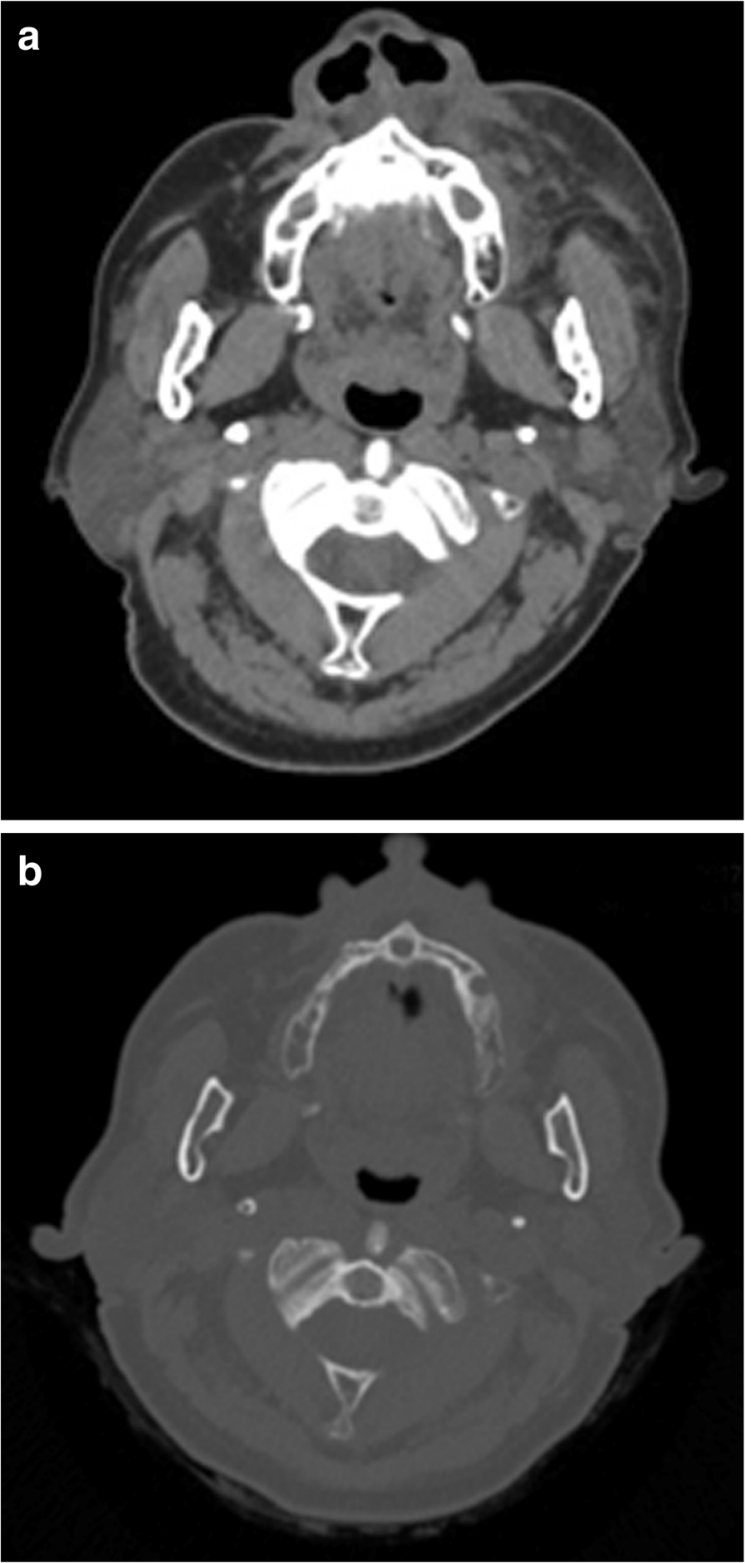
Simple computed tomography image of the neck at the first examination. a Axial image in soft tissue windows (width = 250, center = 30); widespread inflammation with adipose tissue opacities in the left cheek. b Axial image in bone windows (width = 2000, center = 400); a transparent image is seen in the apical region of tooth No. 25 in the left maxilla
